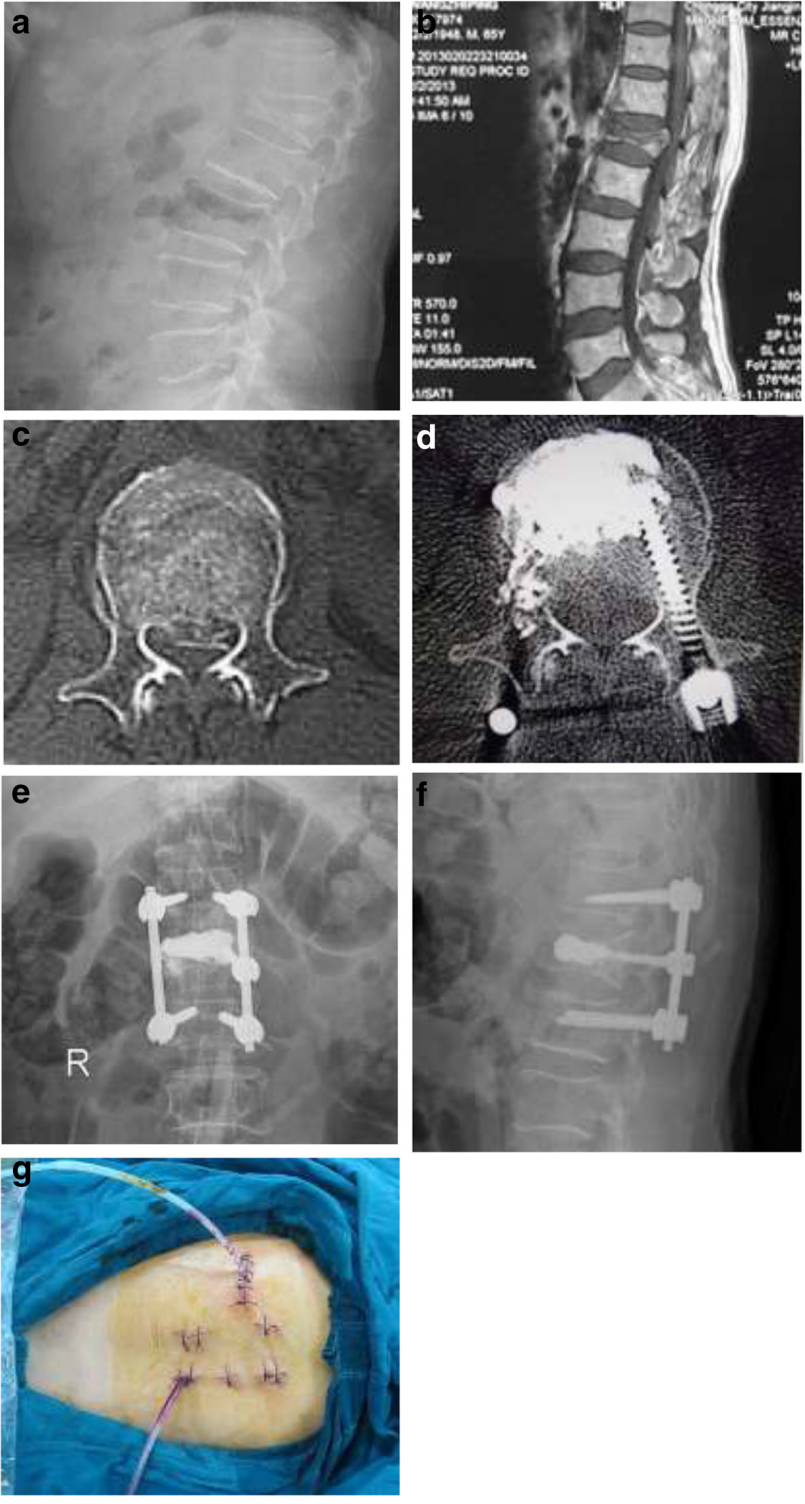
Images obtained in a 69-year-old man who underwent direct decompression followed by percutaneous short-segment fixation and vertebral augmentation: (a) Preoperative lateral X-ray; (b) Preoperative sagittal-sectional MRI; (c) Preoperative cross-sectional CT; (d) Postoperative cross-sectional CT; (e) Postoperative anteroposterior X-ray; (f) Postoperative lateral X-ray; (g) Transverse incision next to erector spinae.
